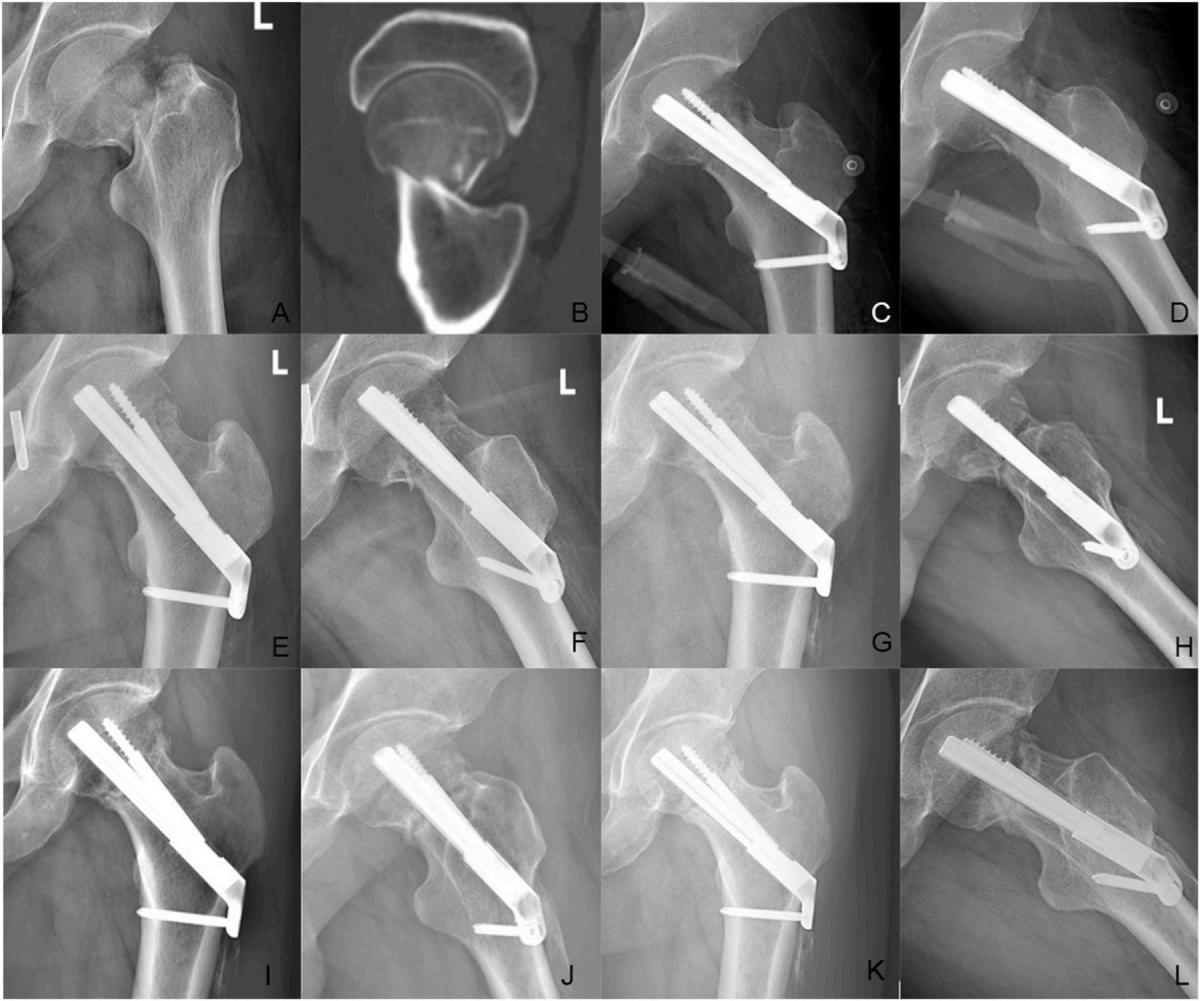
Case presentation: a 35 years old man with left femoral neck fracture fixed with FNS. (A,B) Preoperative anteroposterior radiograph and CT scan showed the left FNF with posteromedial cortex fracture. (C,D) The anteroposterior and lateral X-ray with FNS 1 day after surgery; (E,F) The anteroposterior and lateral X-rays 1 month after surgery; (G,H) The anteroposterior and lateral X-rays 3 months after surgery; (I,J) The anteroposterior and lateral X-rays 6 months after surgery; (K,L) The anteroposterior and lateral X-rays 12 months after surgery.
